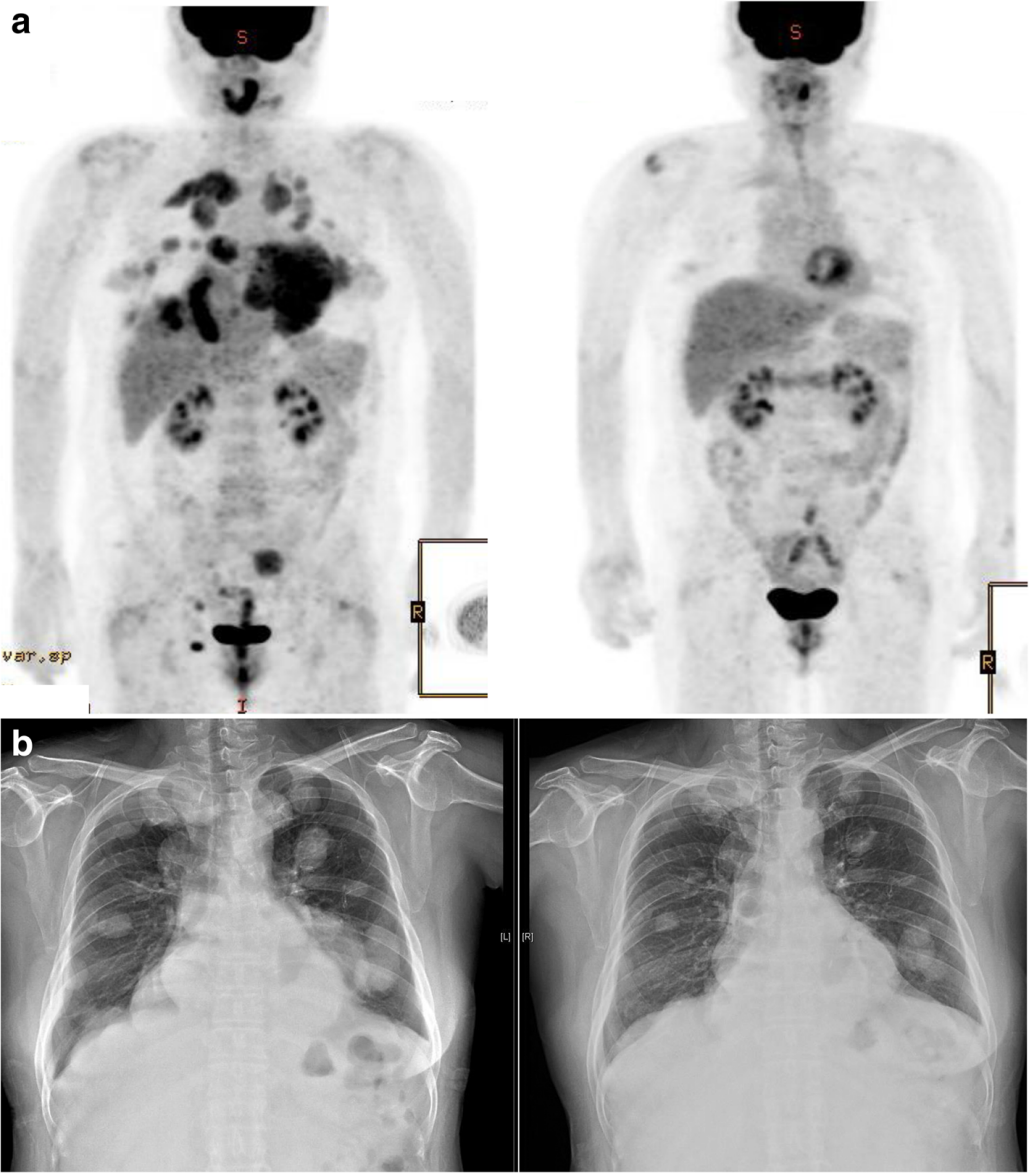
Response to pazopanib therapy in case 1. a. PET-CT at baseline and after 3 months of pazopanib treatment. b. Chest radiograph at baseline and after 4.5 months of pazopanib treatment.
